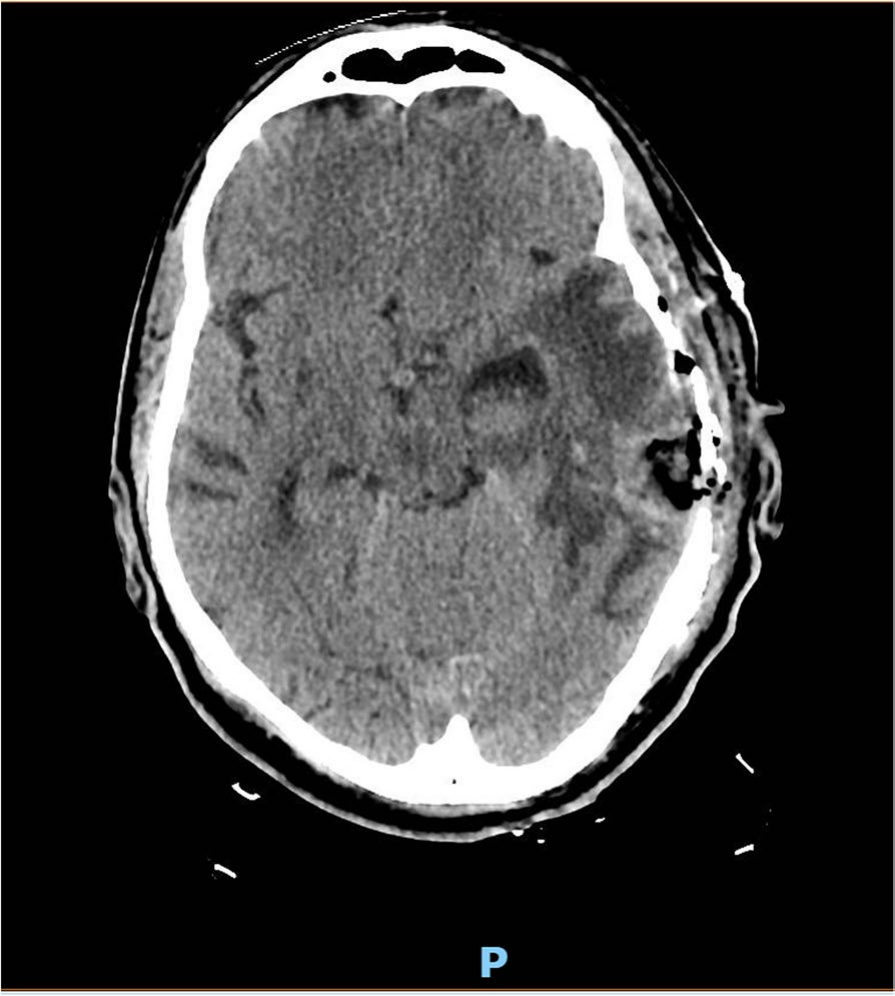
Brain CT-scan, showing left temporomandibular abscesses of 3.9 × 1.8 cm (lateral) and 2.4 × 1.5 cm (medial) respectively with hyperdense margins on baseline scans and post-contrast enhancement
